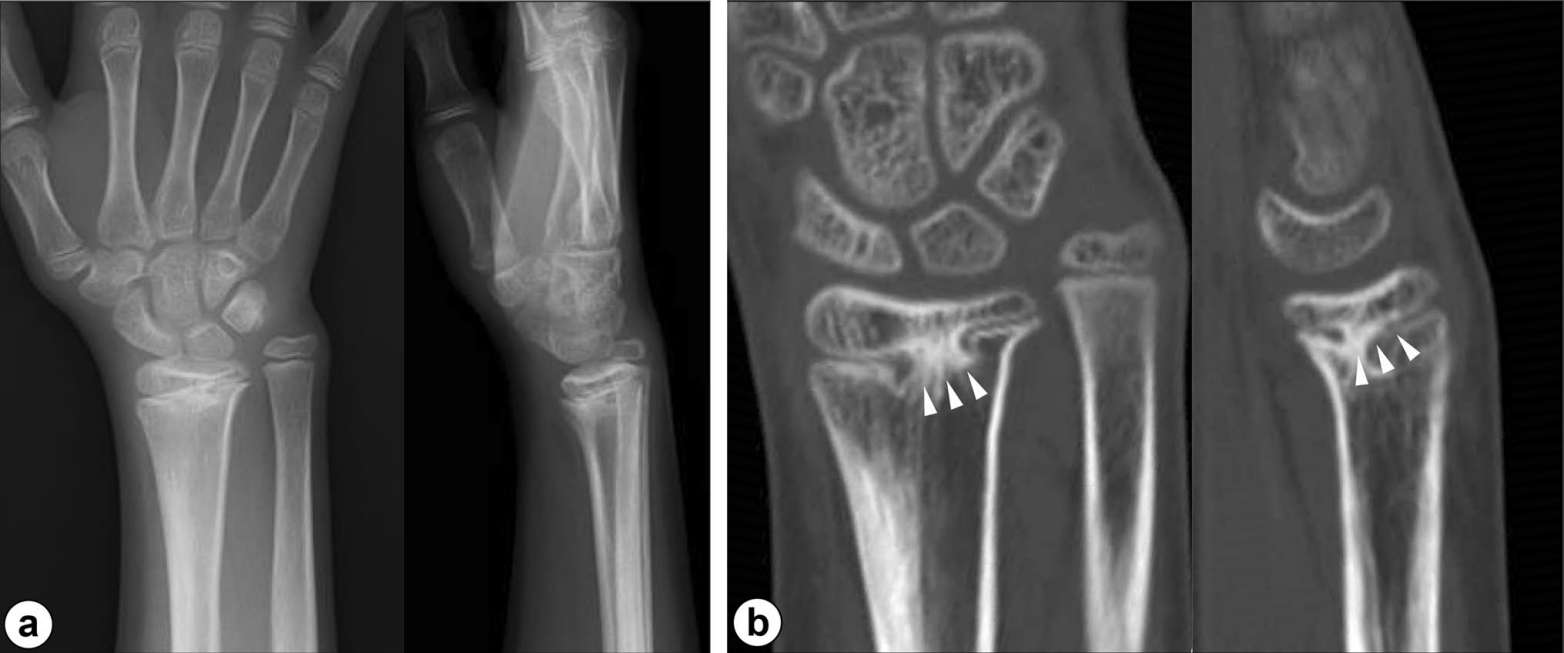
Image findings a year after injury: a anteroposterior (left) and lateral (right) radiographs; and b coronal (left) and sagittal (right) views of the CT scans showing the physeal bar (arrowhead) at the center of the growth plate
